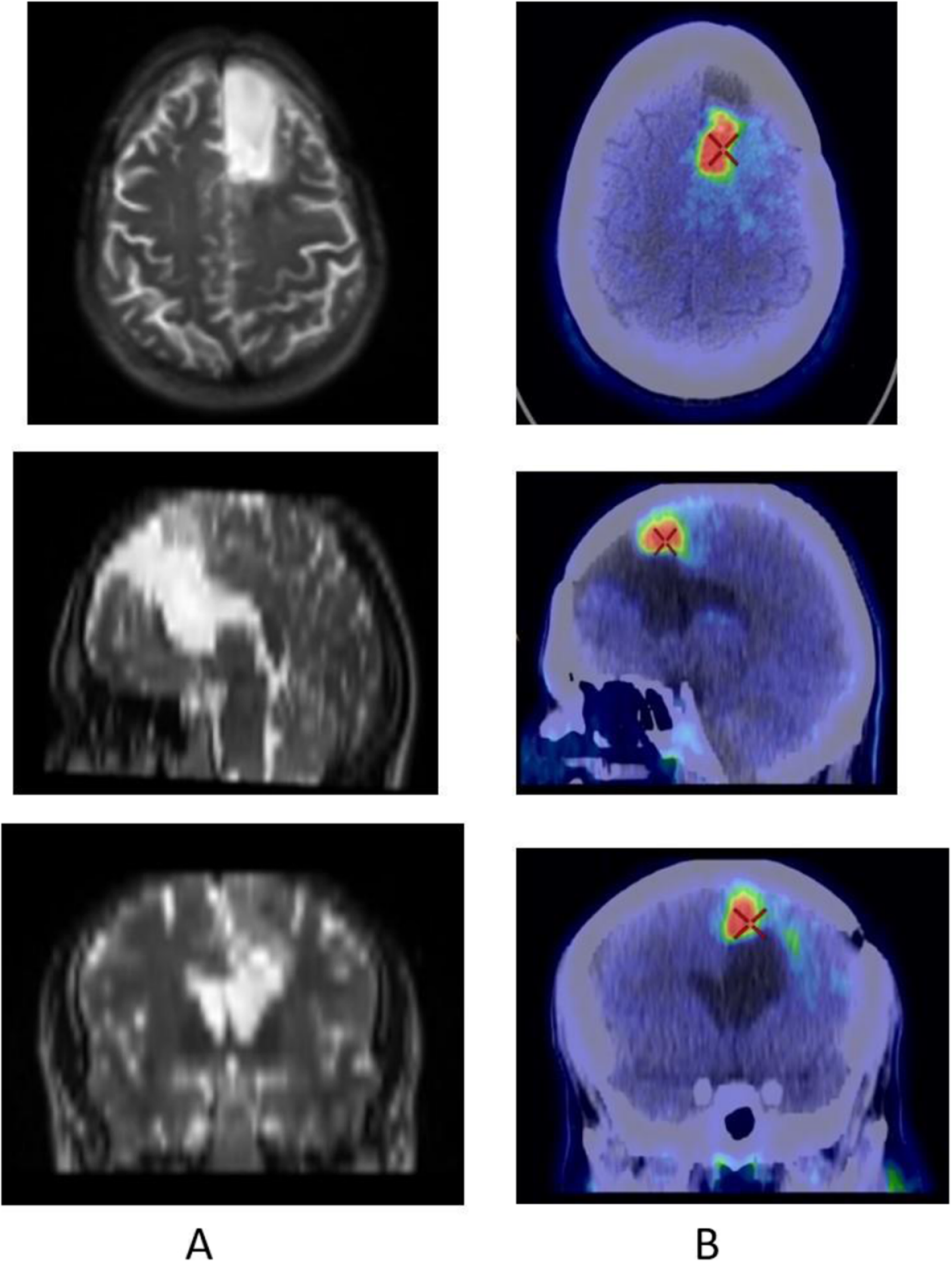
^11^C-Methionine, suspicion of recurrence. *Clinical history*: 41 y.o. male. Left frontal grade III oligoastrocytoma partially removed and treated with radiotherapy and chemotherapy. Asymptomatic for 3 years until relapse. After chemotherapy, MRI showed tumour growth, nodule in external surgical cavity wall, multiple Gd positive foci in left frontal lobe and a smaller one right anterior parasagittal. It is not possible to differentiate between gliosis or relapse (a). *PET/CT findings*: intense ^11^C-Methionine uptake in left frontal lobe related to tumour relapse, which extends to adjacent white matter (b)
